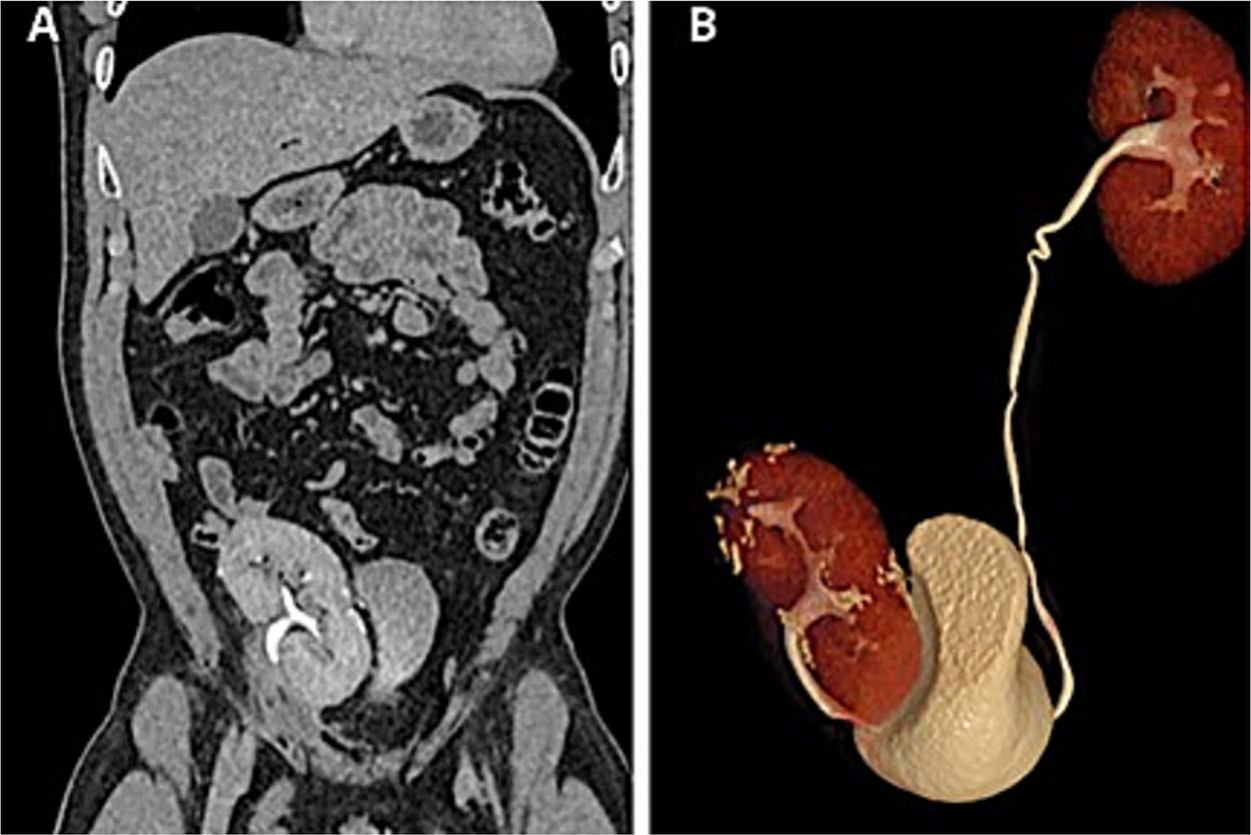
Postoperative CT scan. (A) Autotransplanted right kidney in the iliac fossa demonstrating symmetric contrast excretion and no residual lesions. (B) Normal calyceal morphology and nondilated pelvis; ureters with normal course and caliber.
